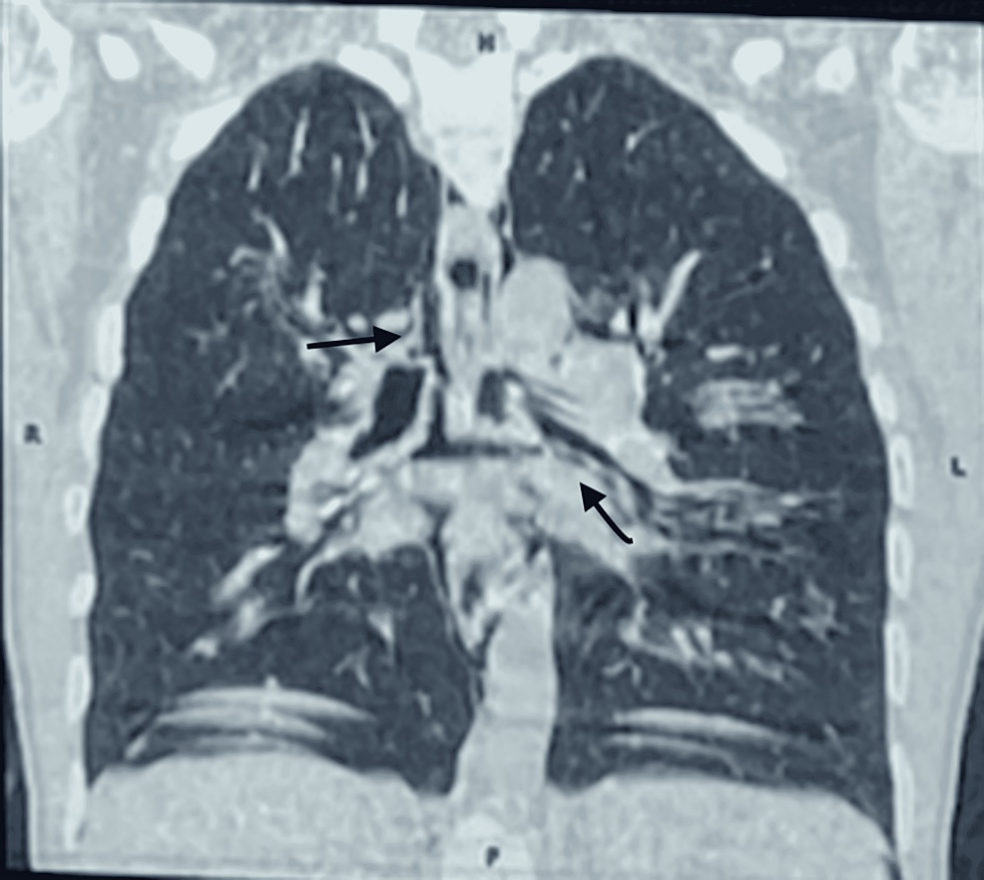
Thoracic CT scan demonstrates a massive pneumomediastinum. The CT scan image confirms air collection along the perivascular connective tissue, the Macklin effect in the peripheric area and the perihilar area, and massive pneumomediastinum.
